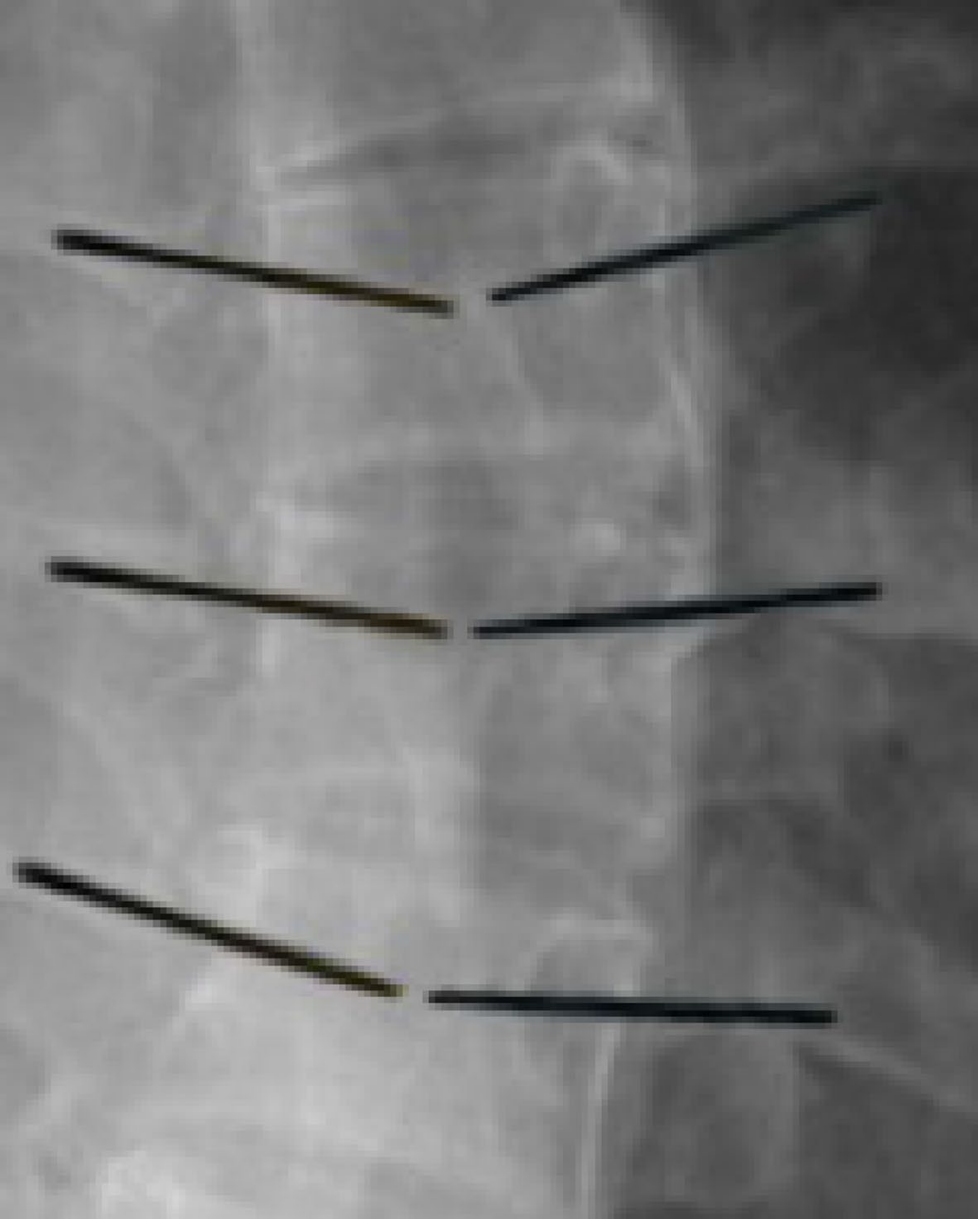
The template was successful applied in vivo trials. The postoperative CT evaluation (performed after each trial session) showed an error less than 1 mm in 94% of the cases and between 1–2 mm in 6% of the cases
